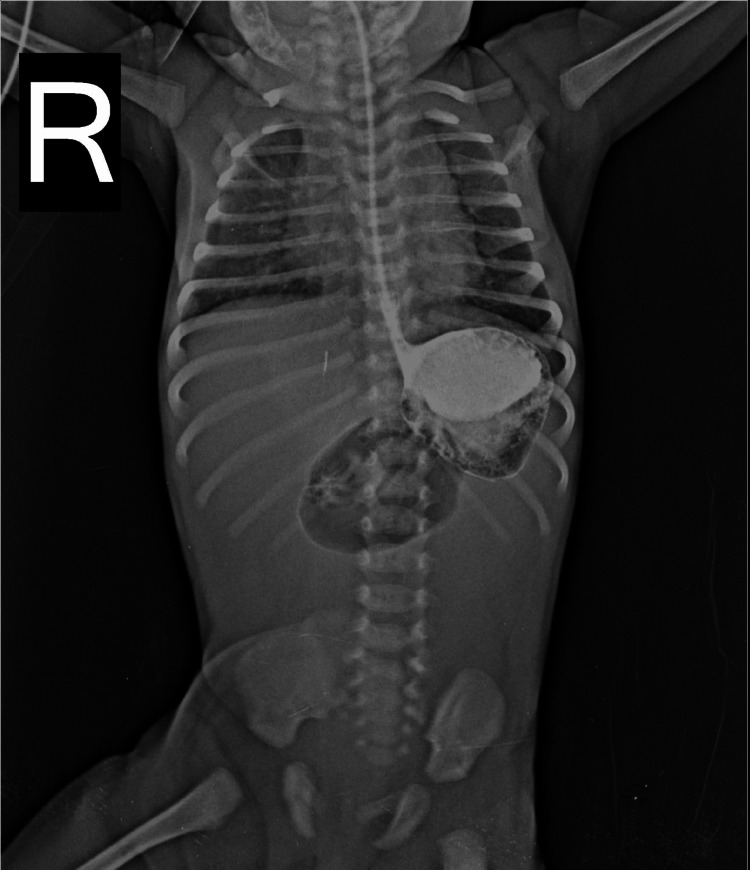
X-ray - erect abdomen (Case 2) A significant radiopaque structure in the right upper quadrant.
